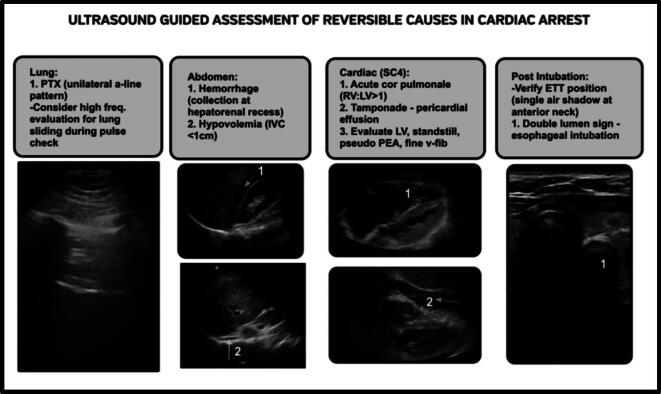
SBUH POCUS protocol during cardiac arrest: views of bilateral anterior chest wall; right upper quadrant; sub-xiphoid or apical four chamber, anterior neck are included with varying pathology.
